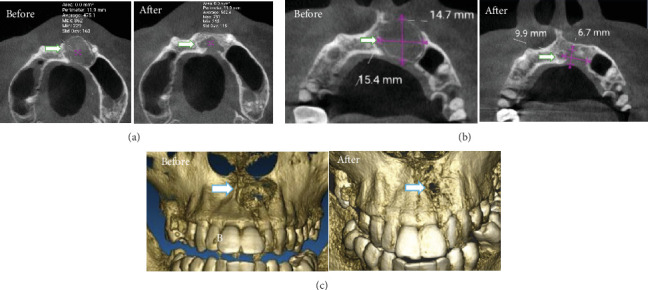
CBCT comparison before and 6 months after surgery. (a) X-ray mode from axial view shows bone density increased 6 months after surgery. (b) The size of the defect area, buccal–palatal expansion, and mesial–distal expansion from axial view reduces after surgery. (c) Bone model shows the cyst defect is still slightly present in Tooth 22, but the surrounding area shows there has been bone regeneration.
